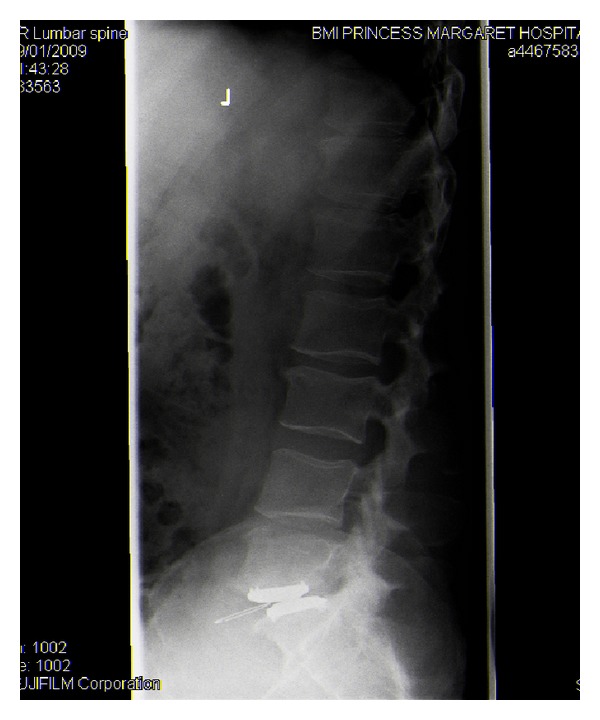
Plain radiograph showing a dislocated polyethylene inlay, but no pedicle fracture is seen.
